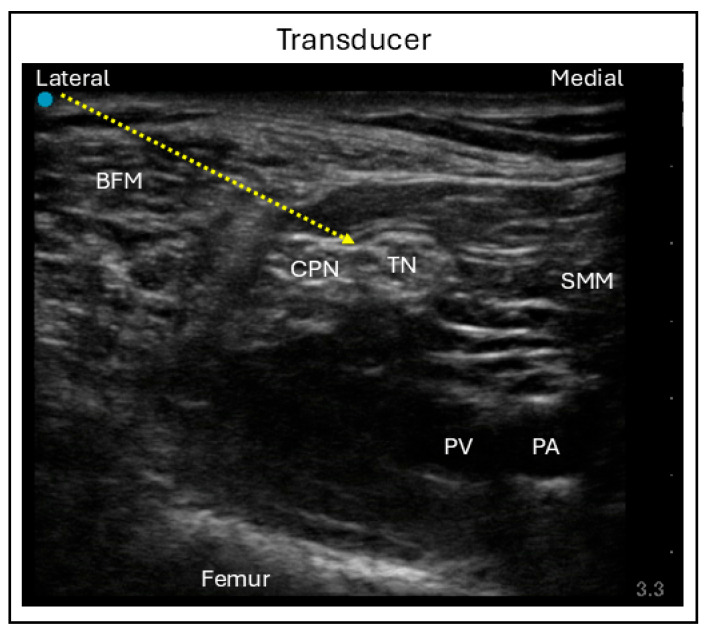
Ultrasound image demonstrating sciatic nerve block using the distal in-plane technique. Dashed line = Needle trajectory; BFM = Biceps femoris muscle; CPN = Common peroneal nerve; TN = Tibial nerve; SMM = Semimembranosus muscle; PA = Popliteal artery; PV = Popliteal vein.
